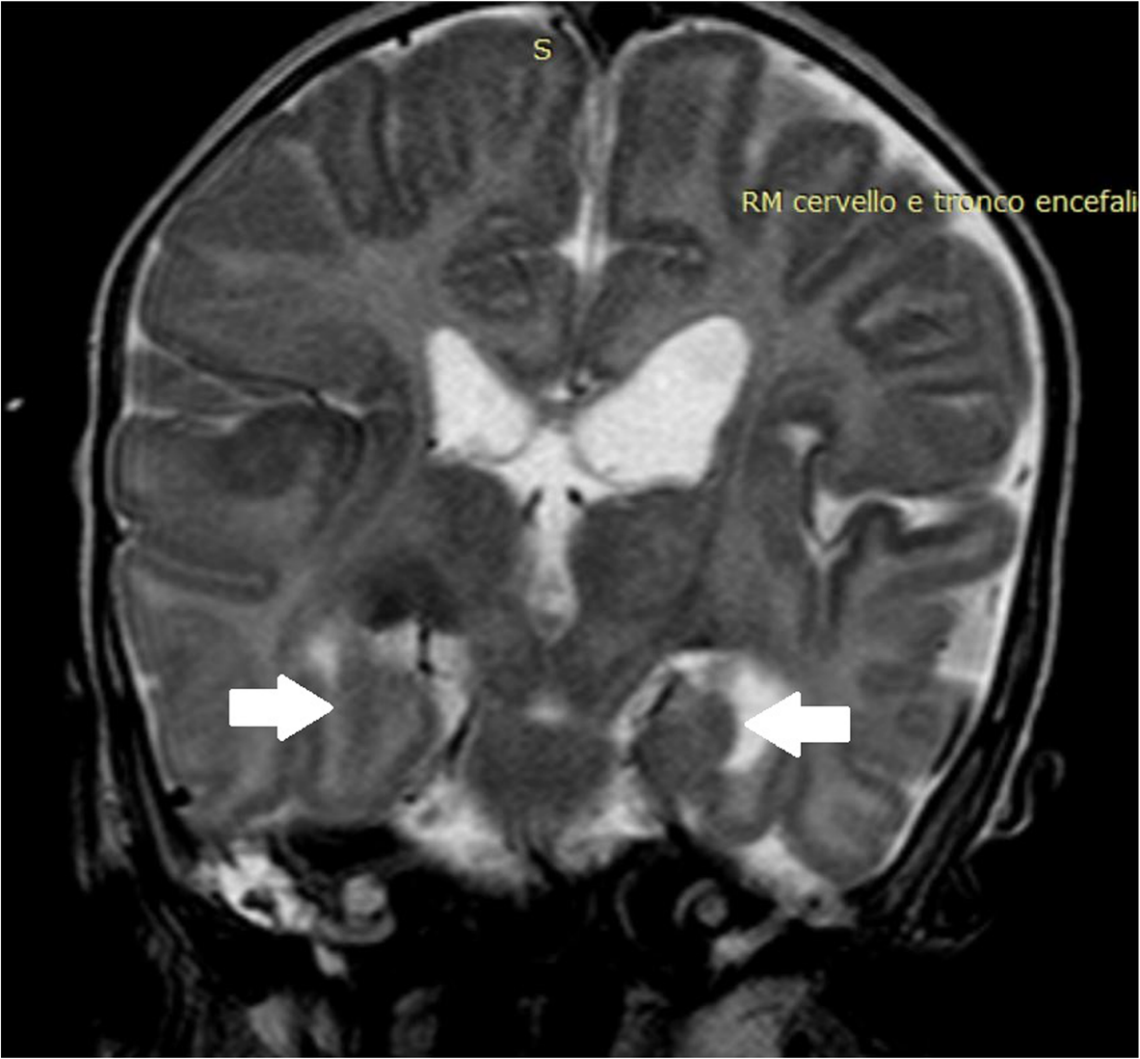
Brain MRI T2 coronal image: incomplete bilateral hippocampal inversion (white arrows) and left lateral ventricle dilatation
